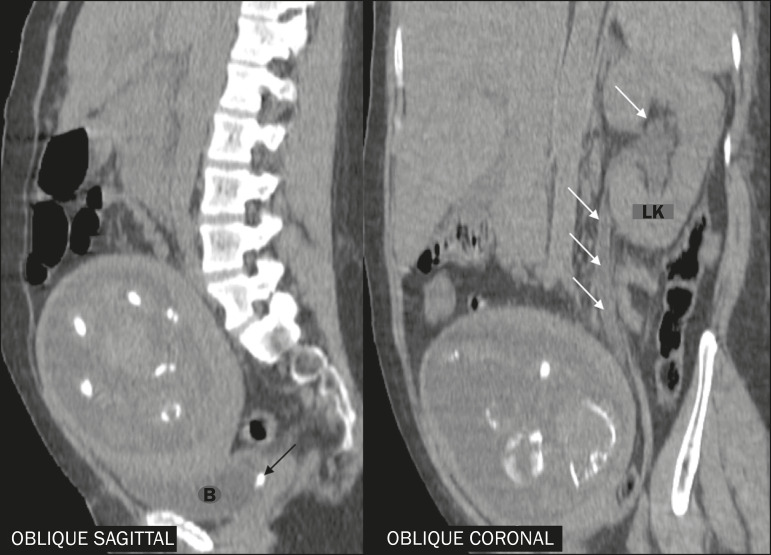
Reconstructions of unenhanced CT scans in the oblique axial, sagittal, and coronal planes, showing ureterolithiasis in a pregnant patient. Note the impacted gallstone in the left distal ureter (black arrow) and the ipsilateral upstream hydronephrosis (arrows). B, bladder; LK, left kidney.
